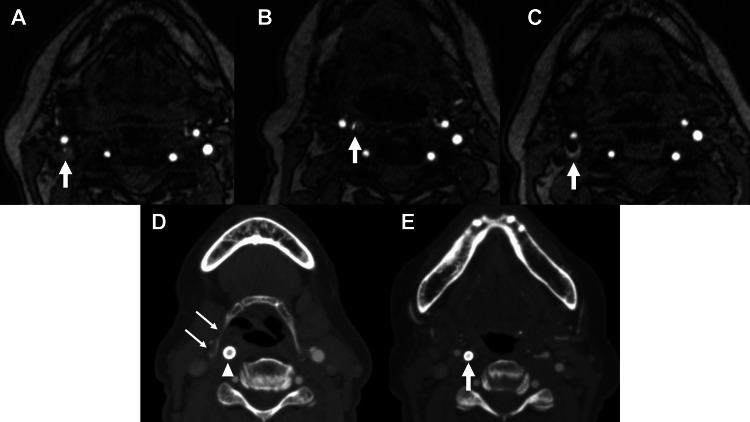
Serial imaging studies showing dynamic position changes of the internal carotid artery before and after stenting Time-of-flight magnetic resonance angiography (TOF-MRA) 2 months before (A), a day before (B), and a day after (C) carotid artery stenting (CAS). The internal carotid artery (ICA) moved into the retropharyngeal space in the MRA the day before CAS and returned to its original position the day after CAS. The arrow indicates the ICA. (D, E) Computed tomography angiography (CTA) 5 days after CAS. The common carotid artery (arrowhead) is medial to the hyoid bone (double arrows), and the ICA (arrow) has also moved medially again.
